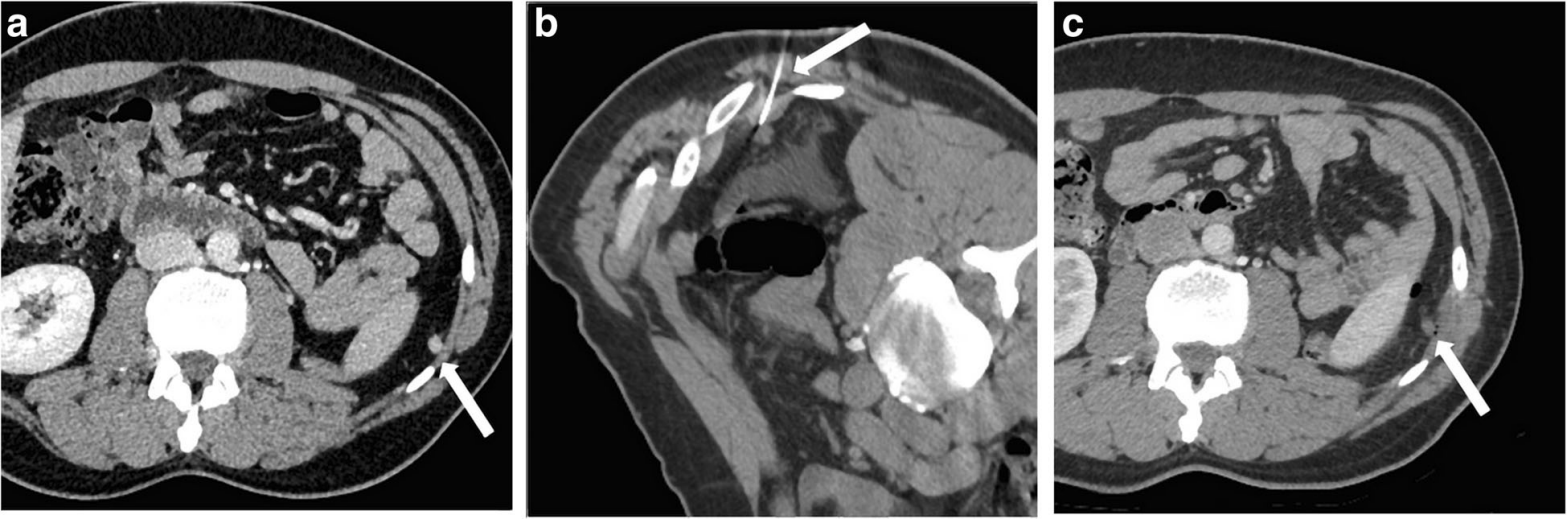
Forty-two-year-old male patient with small left recurrence of renal cell carcinoma. a Contrast-enhanced computed tomography (CT) scan before treatment. A pathological nodule of 8 mm is clearly visualised in the left kidney fossa (white arrow). b Unenhanced CT scan with the patient placed in a prone position. Laser ablation procedure: the needle of the laser device is clearly seen placed insight the pathological nodule during the treatment (white arrow). c Contrast-enhanced CT scan after treatment. The small pathological nodule does not show any contrast uptake demonstrating the effectiveness of the treatment (white arrow)
